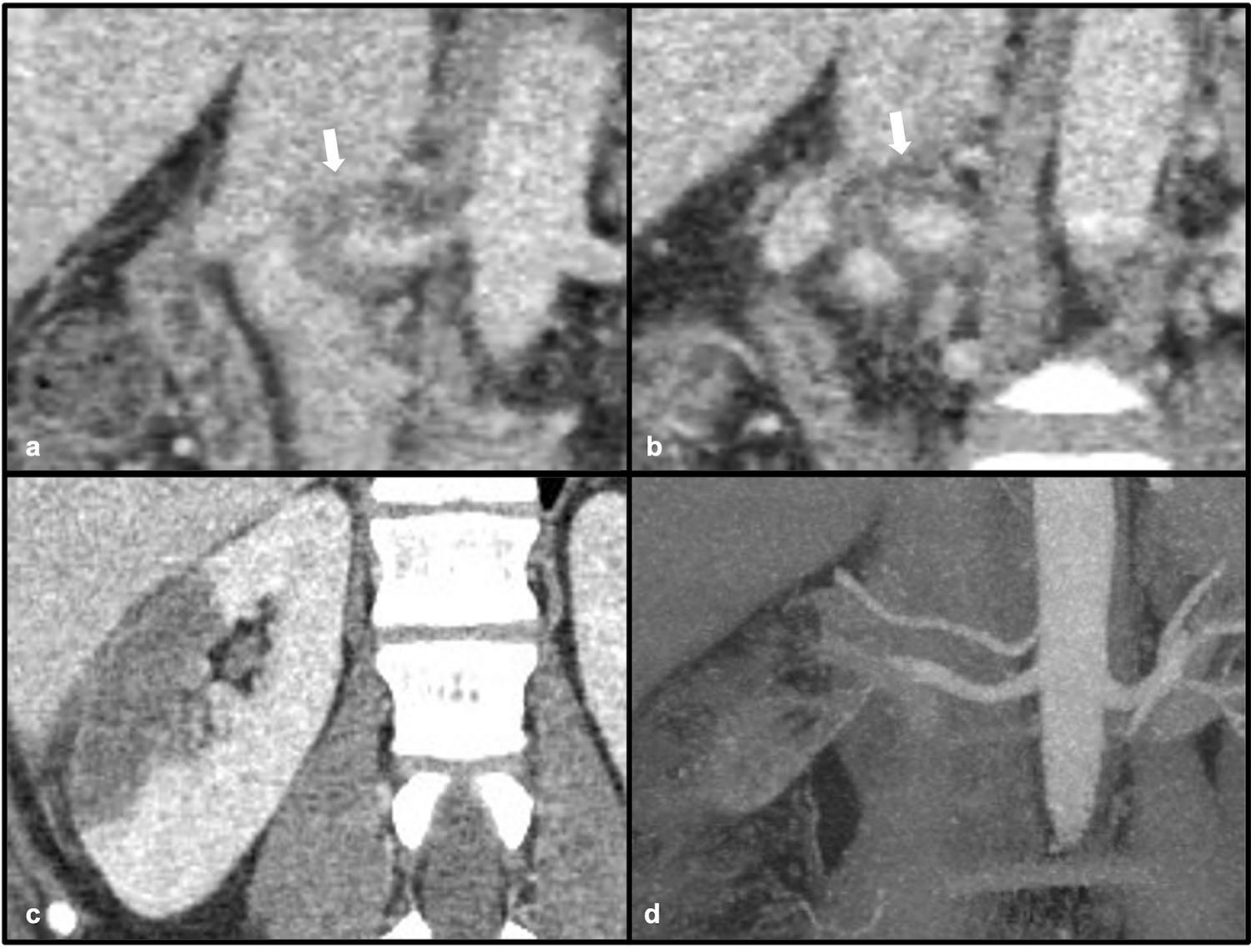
33-year-old male patient with confirmed IgG4 vasculitis. Coronal post-contrast CT images (a) and (b) demonstrate ill-defined, hypoenhancing soft tissue surrounding the right renal artery (white arrows) consistent with inflammation. Coronal post-contrast CT nephrogram (c) demonstrates a large, wedge-shaped cortical defect in the right kidney consistent with infarct. Coronal CT angiogram (d) shows resolution of the inflammation following steroid therapy
